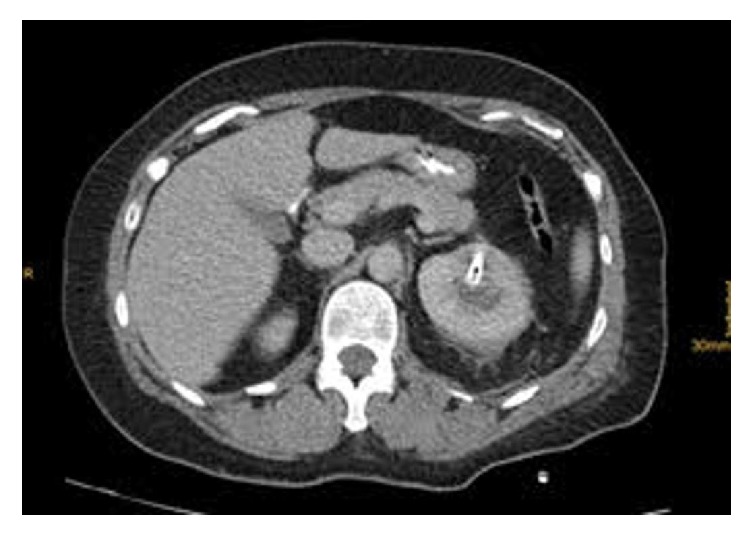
Abdominal CT scan (fourth postoperative day) demonstrating no pathologic findings from pancreas and nephrostomy tube in the left renal pelvis.
